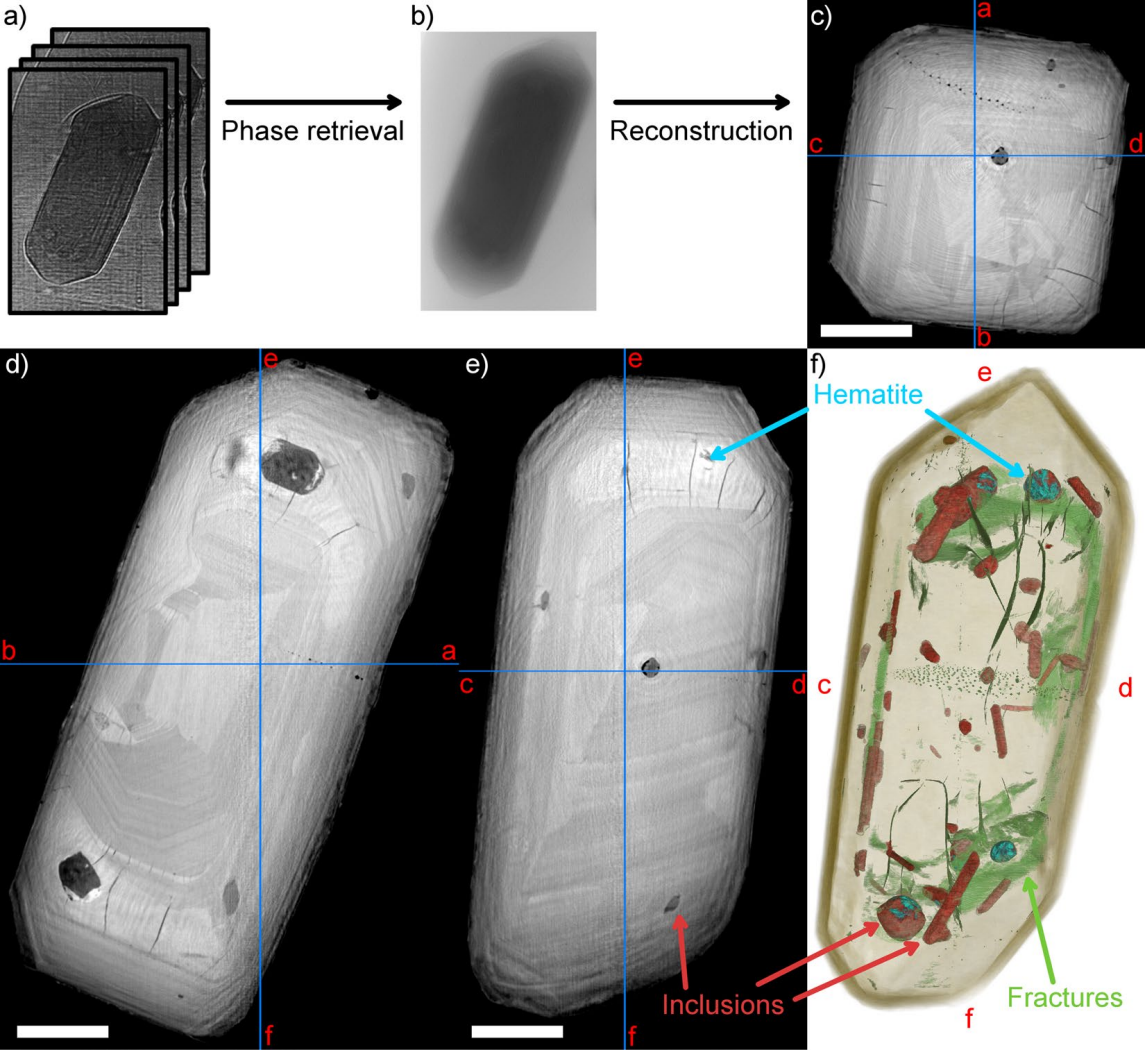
The principle of propagation-based phase contrast tomography and results of zircon 1973A. (a) Untreated projection images. The scan consists of acquiring 4 tomographic scans with varying sample-to-detector distance. (b) One phase map, i.e. projection image which is the result of passing the four images with different propagation distance through a phase retrieval algorithm. Each pixel in the image represents the phase change of the X-rays traversing through the sample. (c–e) Axial (x-y), and two longitudinal (x-z and y-z) slices of the tomographic reconstruction computed based on the phase maps. The blue lines indicate the positions of the orthogonal slices in each. Scale bars are 20 µm. (f) 3D rendering of the whole volume (i.e. projection of the 3D structure to the image plane), showing the zircon matrix in brown, porosities and cracks within the zircon in green, inclusions in red, and the dense Fe-rich phase (hematite, cf. Figs 2 and 5) inside the inclusions in blue. A video showing the zircon from different directions is included as video S1 in the supplementary material.
